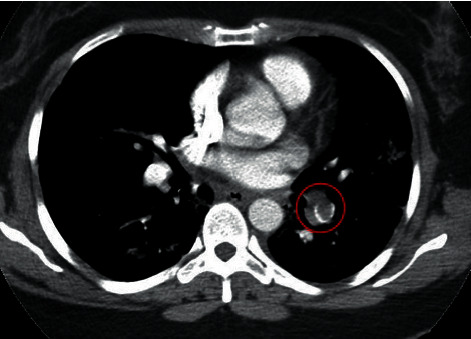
Chest axial CTA image on a vascular window shows a filling defect (circle) in the left lower lobe consistent with lobar and segmental pulmonary embolism.
